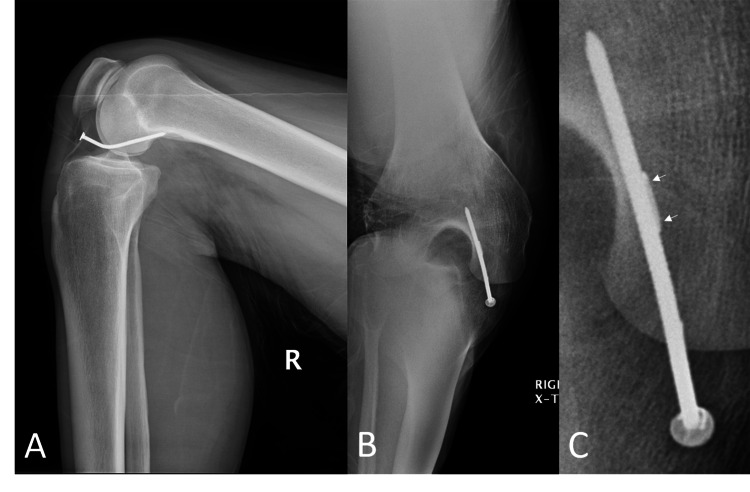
Plain radiography revealed a penetrating nail entering the medial aspect of the knee, just medial to the patellar tendon, and entering the medial femoral condyle (A, B). In magnified view, the nail barb (arrows) is visible (C).
